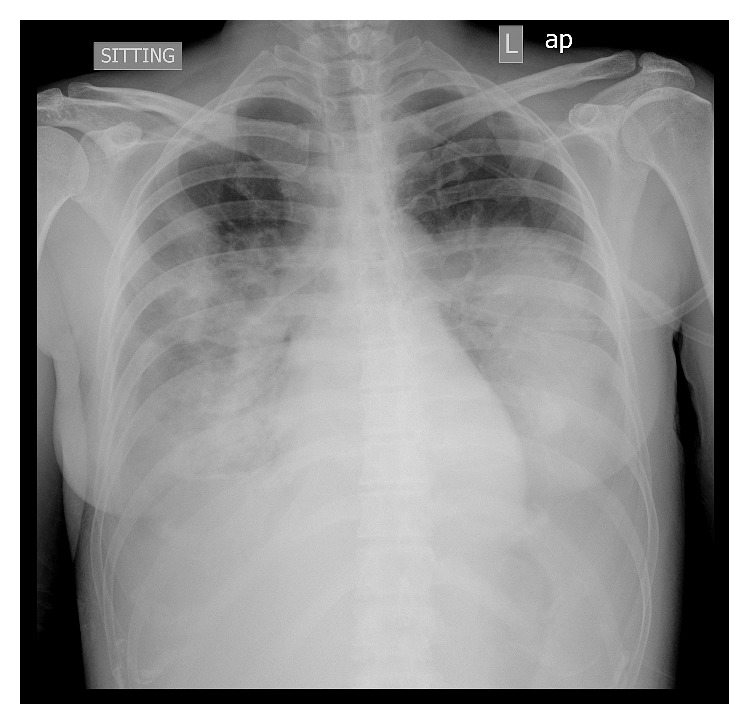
The patient's chest X-ray showing extensive bilateral airspace disease consistent with ARDS.
